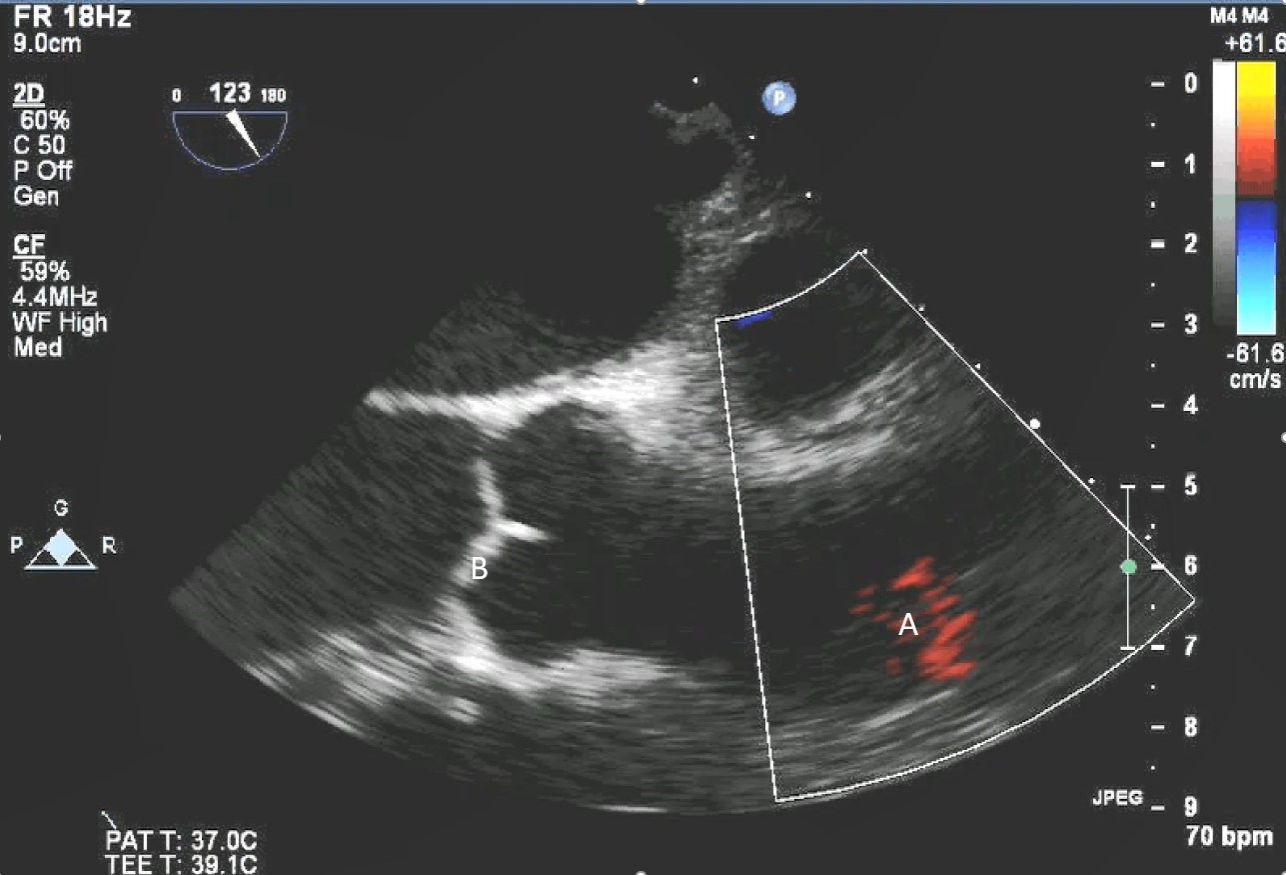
High-esophageal transesophageal image with the LVAD outflow
